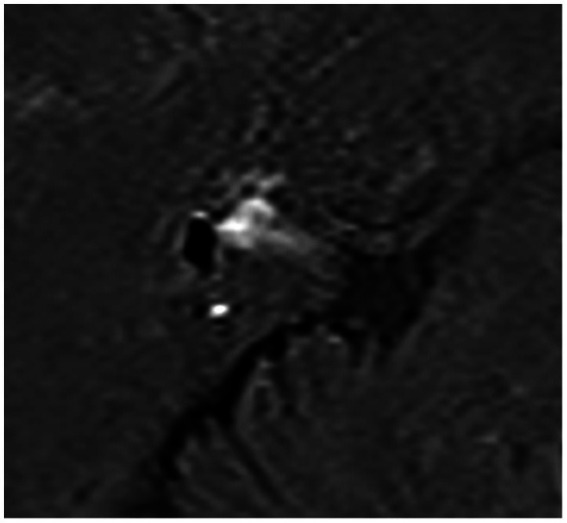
Endolymphatic MRI of a patient with unilateral MD, showing more severe hydrops in the vestibule than in the cochlea.
